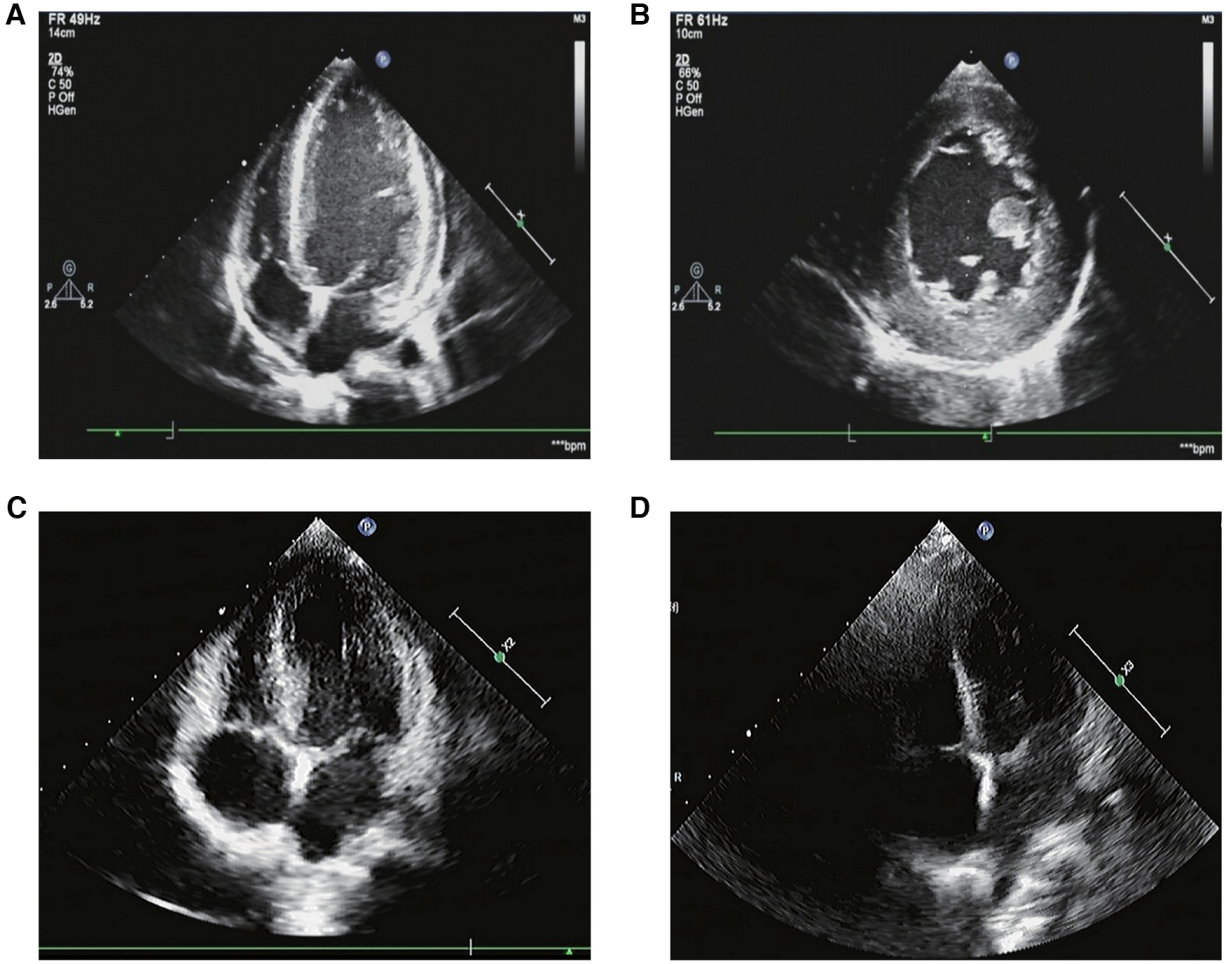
The transthoracic echocardiography. (A,B) The transthoracic echocardiography before the tumor resection. (1) The left atrium and ventricle were both enlarged. The ventricular septum and left ventricular free wall were generally thickened. The thicker left ventricular free wall was situated in the middle of the lateral wall, measuring about 14 mm in thickness, while the thicker ventricular septum was situated in the basal segment of the anterior septum, measuring about 12 mm in thickness. The estimated ejection fraction (EF) was about 40%. (2) Both of the bilateral coronary arteries’ internal diameters were increased, with the left main coronary artery’s diameter measuring 4.1 mm and the right main coronary artery’s diameter measuring 3.0 mm. (3) A fluid dark area was observed in the pericardial cavity, 8 mm in the right ventricular sidewall and 4 mm in the apex. (C) The transthoracic echocardiography 3 months after the tumor resection. The left ventricle was mildly enlarged. The left ventricular wall was slightly thickened. The thicker left ventricular free wall was situated in the middle of the lateral wall, measuring about 9.5 mm in thickness, while the thicker ventricular septum was situated in the basal segment of the anterior septum, measuring about 10.6 mm in thickness. The systolic function of the left ventricle was normal. (D) The transthoracic echocardiography 7 months after the tumor resection. The measured values of the internal diameters of each atrioventricular cavity were normal. Each chamber wall’s thickness and movement were both typical. The systolic function of the left ventricle was normal.
